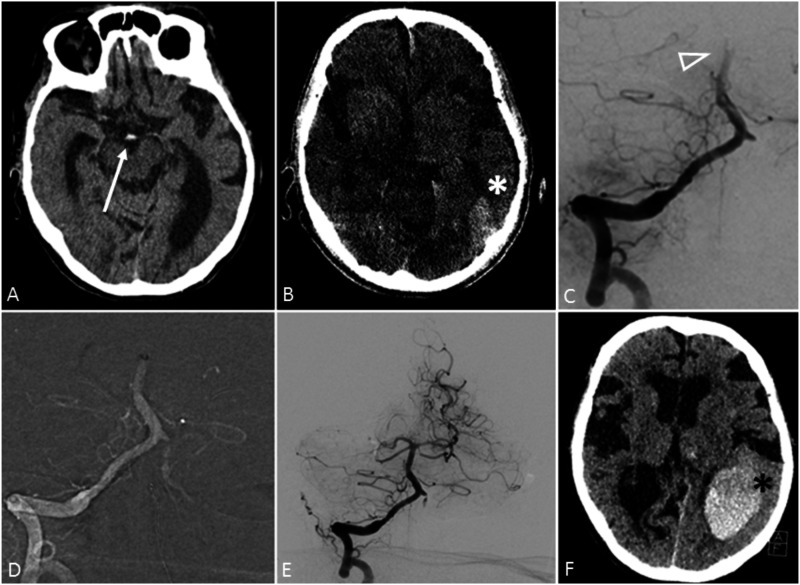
(a) Baseline imaging from an octogenarian woman with basilar artery occlusion and dens artery sign (white arrow) on non-contrast computed tomography. Intravenous thrombolysis was given, and the patient was transferred to a comprehensive stroke center. (b) At the beginning of the endovascular procedure a flat detector computed tomography was done due to deterioration of symptoms (National Institute of Health Stroke Scale=22) with evidence of an intraparenchymal haemorrhage in the left occipital lobe (white asterisk). At this timepoint, alteplase was already administered completely. (c)–(e) Mechanical thrombectomy was done with successful recanalization of the basilar artery. The right posterior cerebral artery was chronically occluded. (f) Computed tomography 24 h later showed an aggravation of the intracranial haemorrhage (black asterisk). The patient died due to respiratory failure during the hospital stay.
